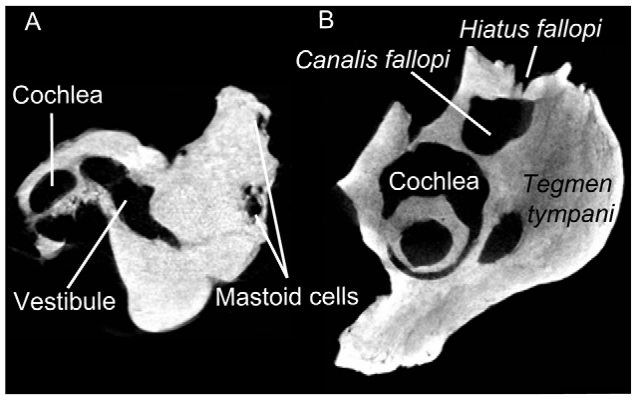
CT-radiography of CBI-1-542. A: slide at the level of the *pars mastoidea* showing cancellous cells in the mastoid apophysis; B: slide at the level of the *hiatus fallopi* showing the communication between the *hiatus fallopi* and the *canalis fallopi*.
